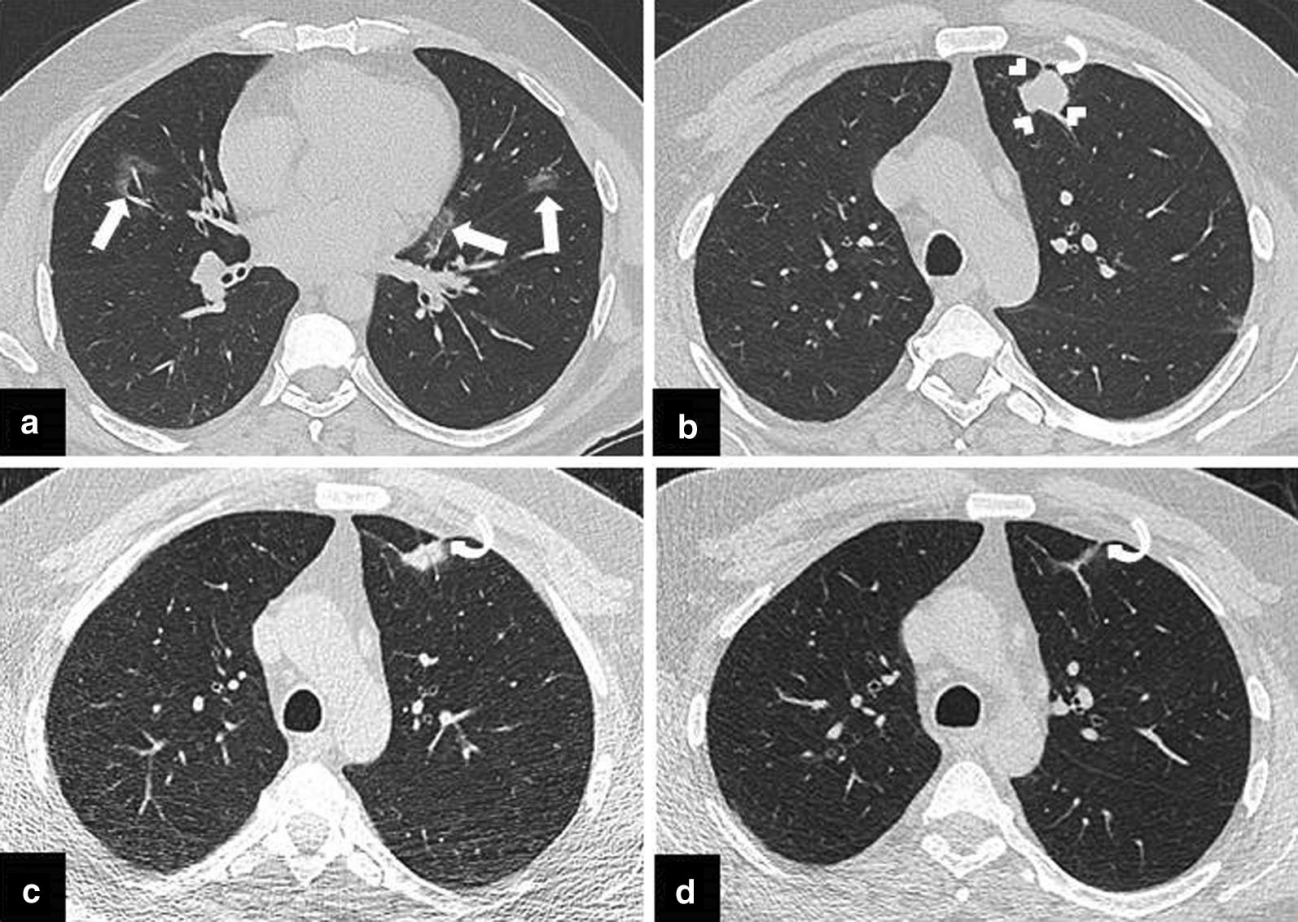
a, b The initial chest CT scan obtained following PCR test positivity for COVID-19 infection, revealed a few patchy areas of ground glass opacity (GGO) in both lungs (arrows) compatible with COVID-19 pneumonia. An irregularly shaped solid nodule 2 cm in diameter in left upper lobe of the lung was also noted (arrowheads). Percutaneous transthoracic core needle biopsy was scheduled due to suspicion of primary lung cancer. c CT scan obtained prior to biopsy procedure demonstrated significant size reduction of the nodule. Therefore, biopsy was not performed. d Follow-up CT scan obtained 3 months later demonstrated complete resolution of the nodule. A pleural tag which became more apparent following resolution of the nodule (curved arrows, b–d) raised the suspicion of COVID-19 triggered focal organizing pneumonia
